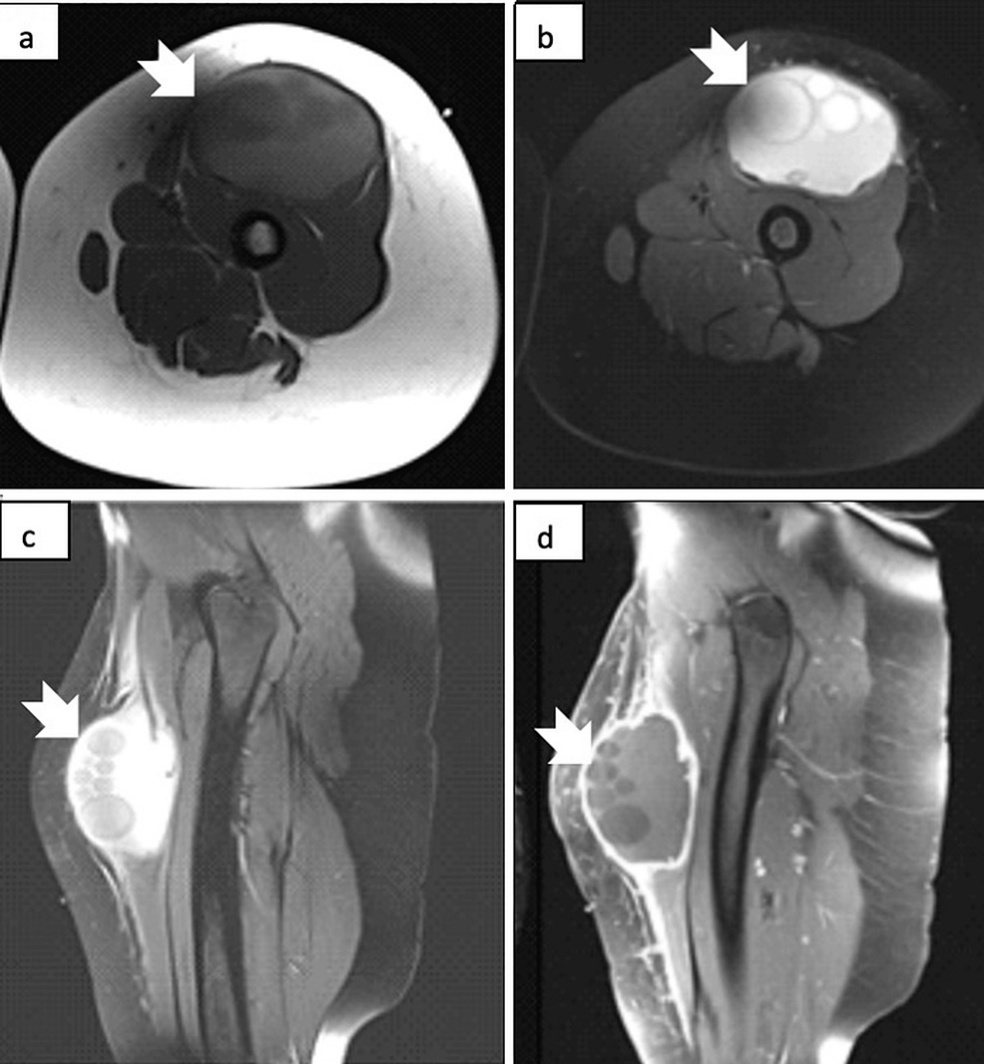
MRI demonstrating the lesion in the left thigh (a) Axial T1-weighted MRI demonstrating multiple daughter cysts as hypointense signals (arrows), relative to surrounding intracystic fluid (white arrow). (b) Axial fat-suppressed T2-weighted image showing cyst formations in the thigh; the daughter cystic wall has a hypointense signal (white arrow). (c) Sagittal fat-suppressed T1-weighted image showing cystic formations in the thigh; the daughter cysts have a hypointense signal (white arrow). (d) T1-weighted image with fat suppression reveals peripheral contrast enhancement after gadolinium injection.
